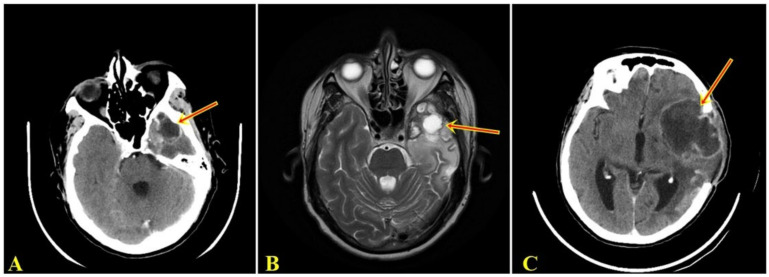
(A). Computed tomographic examination showing space-occupying mass, supratentorial, intranevraxial, with polylobular contour, with peripheral contrast, inhomogeneous structure, and a dense peripheral iodophilic component with axial diameters 42/34 mm, located left temporal that associates perilesional digitiform edema determining the asymmetry of the ventricular system. (B). MRI examination denotes a left temporal supratentorial tumor mass, with polylobate contour, in hypersignal T2 with inhomogeneous contrast, with axial diameters 49/44 mm, surrounded by edema. (C). Computer tomographic examination shows intraaxial lesion, with polylobate contour, with peripheral contrast, with inhomogeneous structure, with axial diameters of 75/57 mm, located left frontal with digitiform edema, with displacement of midline structures by 4 mm to the right, with slight proportion by postoperative bone breach.
